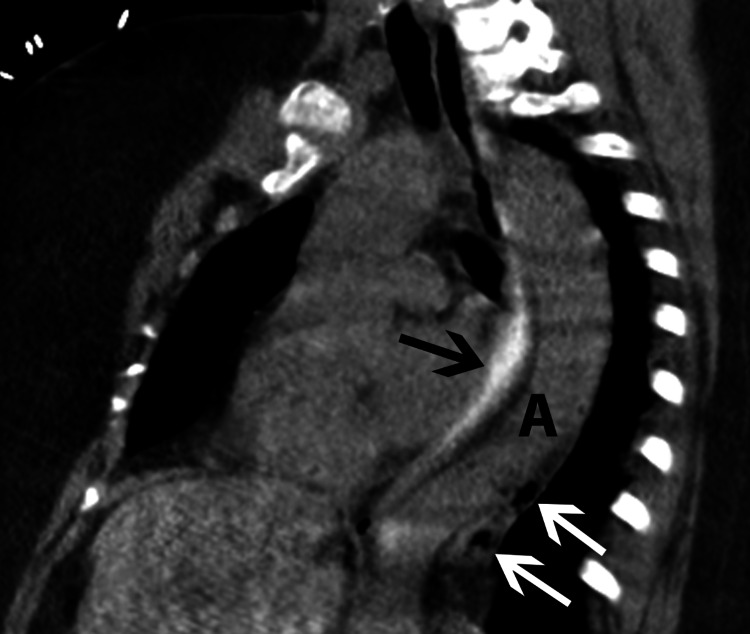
Reformatted oblique sagittal image from subsequent CT esophagram shows contrast in esophagus (black arrow) with no evidence of esophageal leak. Air bubbles are present in the posterior mediastinum (white arrows) posterior to the descending thoracic aorta (A).
